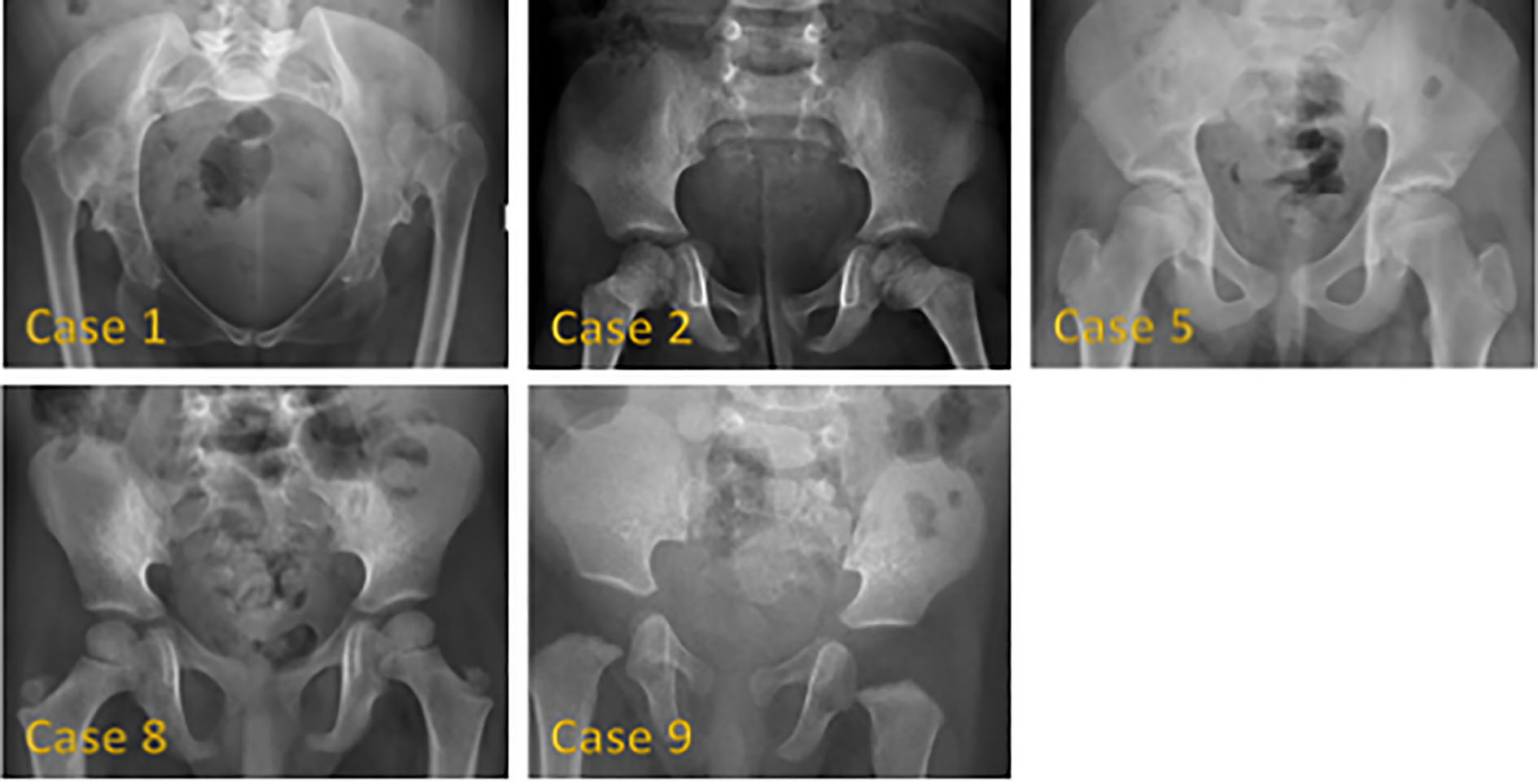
Pelvic radiographs of 5 patients with 3M syndrome. No obvious abnormality was found in Case 2. The pelvic measurements of Cases 5, 8 and 9 are smaller than their peers. Cases 8 and 9 showed a narrow triangular pelvis. Cases 1 and 5 showed a wide pelvis. Case 1 also showed severely dysplastic hip joints, pseudoacetabili formation, and superiorly displaced bilateral femoral heads.
